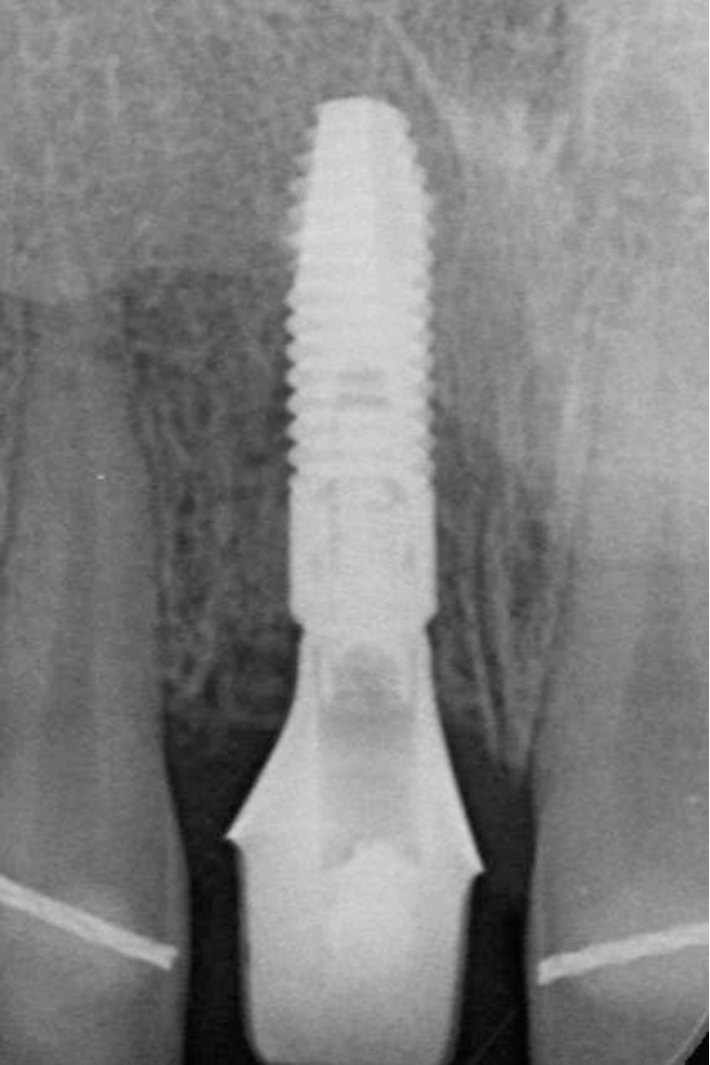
Radiographic view of implant in preserved ridge and temporary restoration.
